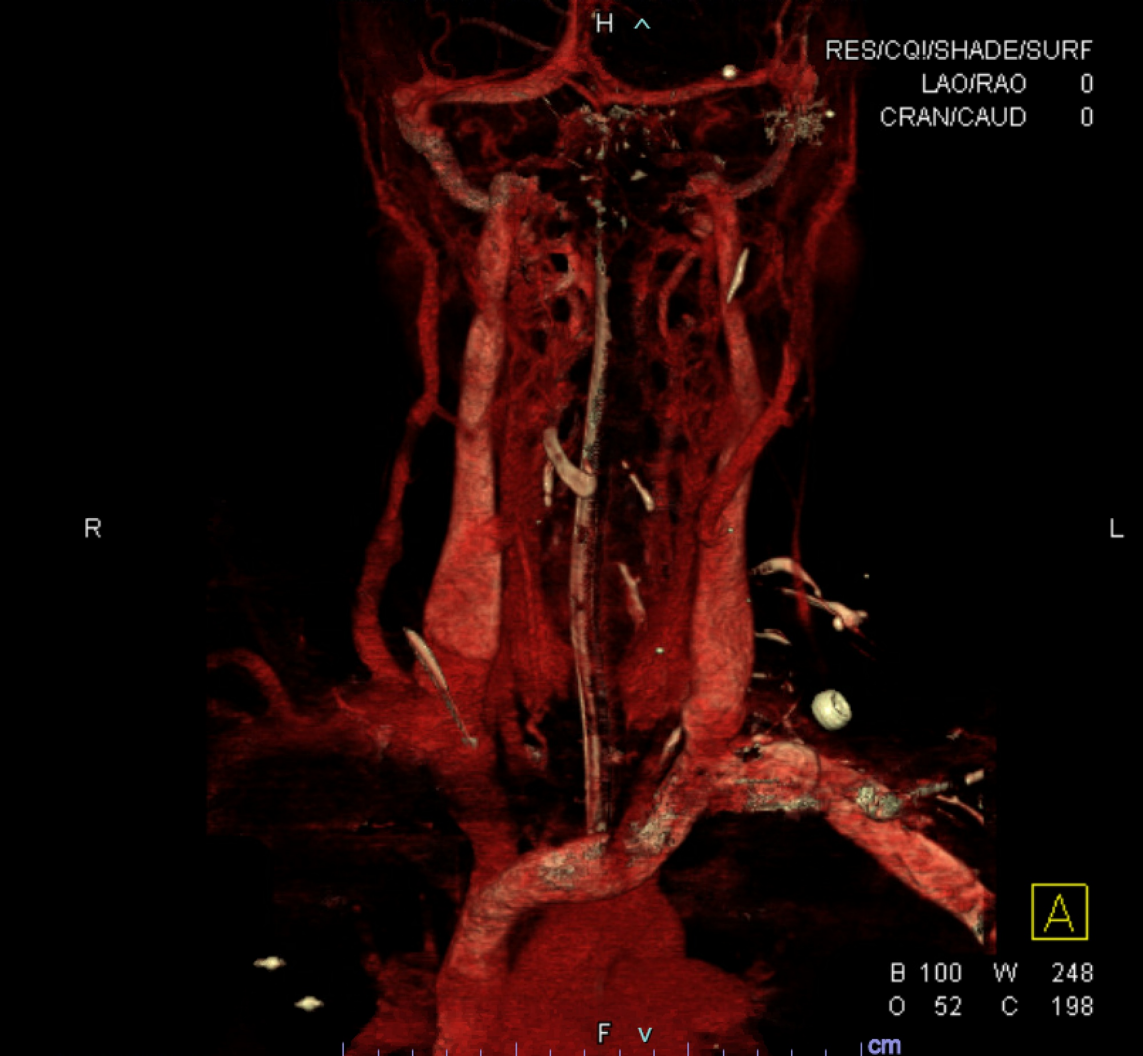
CTA head and neck coronal 3D reformats
